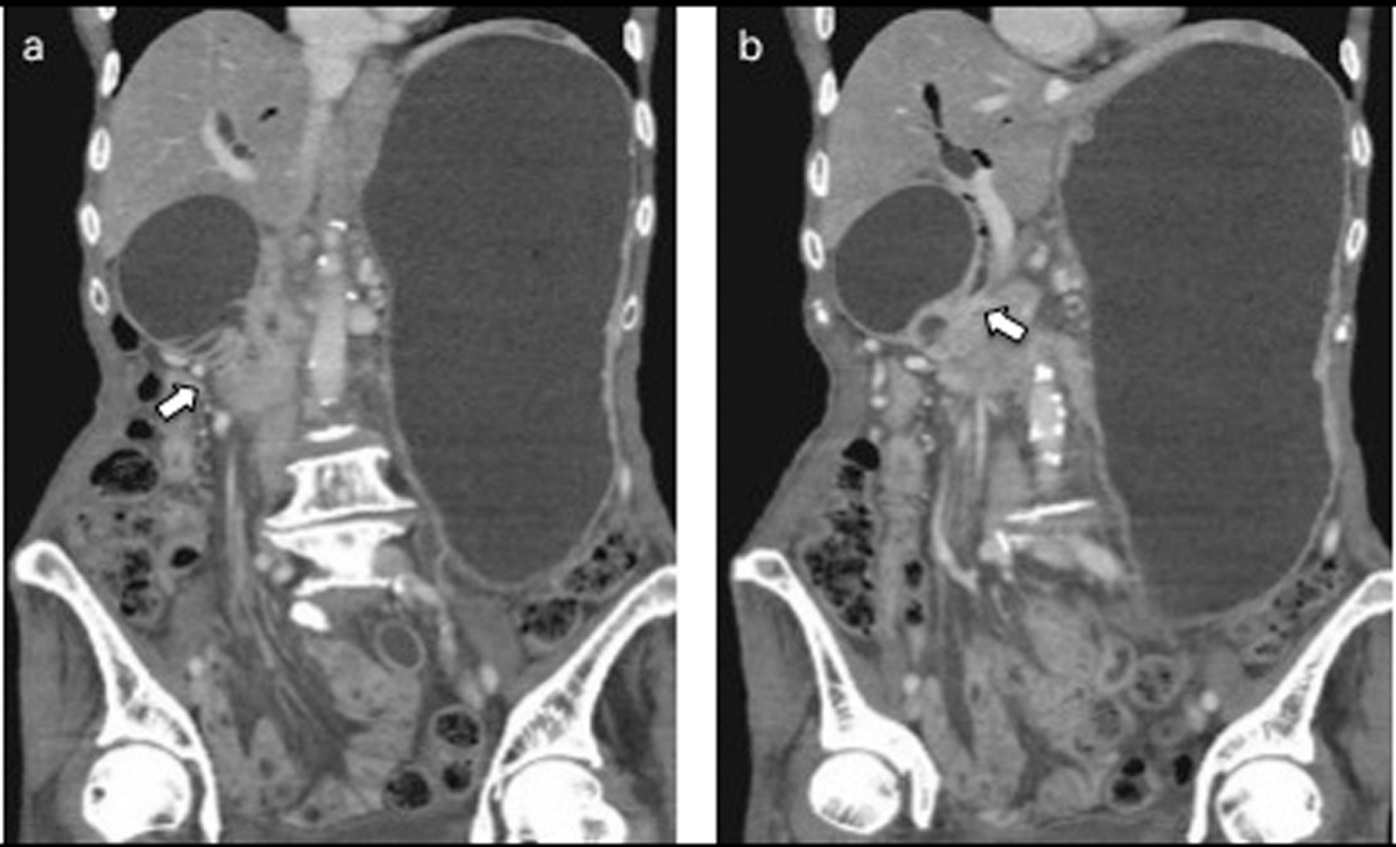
a: Abdominal CT after 7 months of insertion of the metal stent revealed tumor growth in the pancreas and marked distension of the stomach and an obstruction of the descending part of the duodenum by the tumor (arrow). b: The SEMS was not present in the CBD (arrow).
